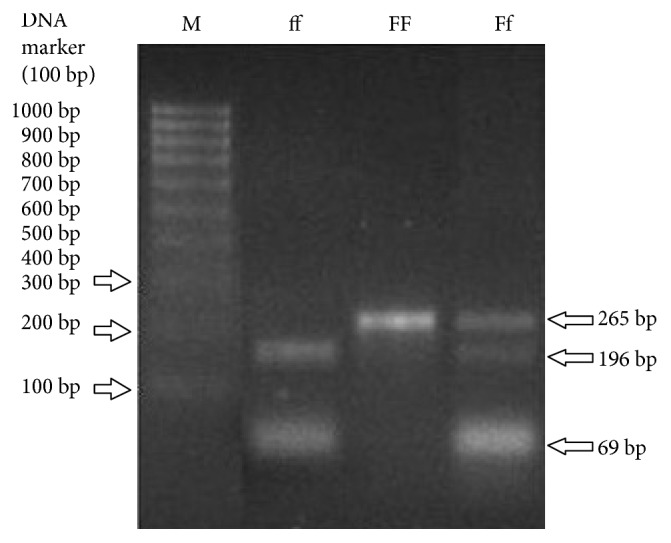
FokΙ* digestion (SNP C/T) in exon 2: Restriction site presence is designated by “f,” and absence is designated by “F.” ff (CC), for example, a 196 bp and 69 bp; Ff (CT), for example, 265 bp, 196 bp, and 69 bp, bands; FF (TT), for example, 196 bp and 69 bp bands.
